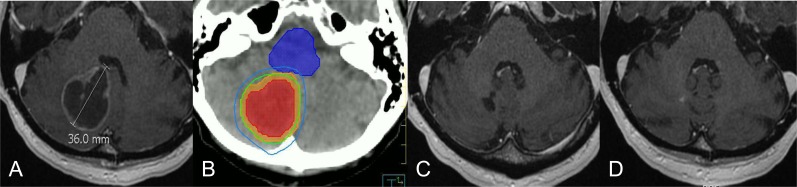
Selected case of large brain metastases treated with hypofractionated stereotactic radiation A. MRI of 3.6cm right cerebellar metastases from lung cancer. B. Highly conformal treatment plan with the 100% (green line) and 80% (blue line) isodose lines wrapping tightly around the gross tumour (red colorwash), and planning target volume (orange colorwash). The brainstem (blue colorwash) is spared from high dose. (B) MRI 2 months post completion of radiation (30Gy in 5 fractions). C. Complete resolution of the tumour at 1 year.
